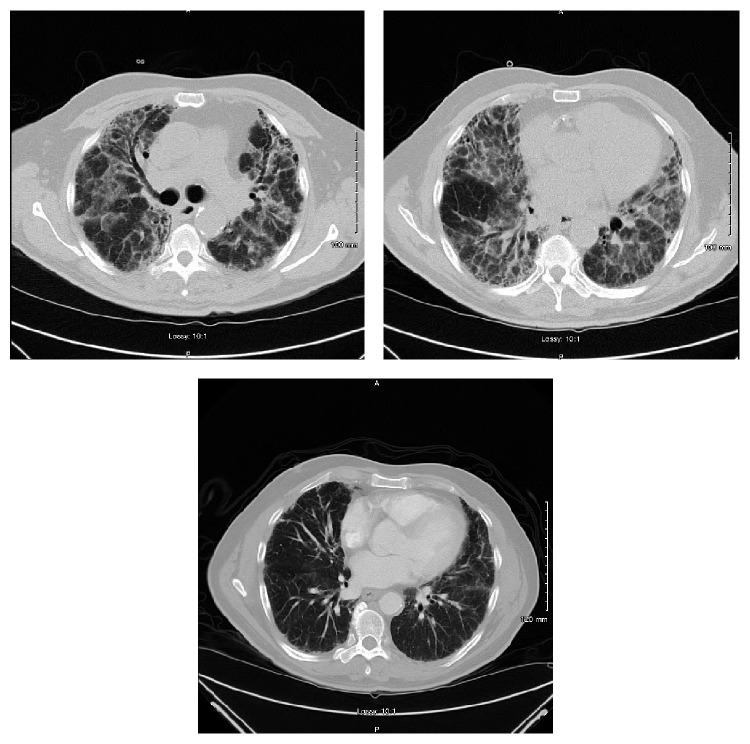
CT scan of the chest showing extensive honeycombing consistent with UIP.
